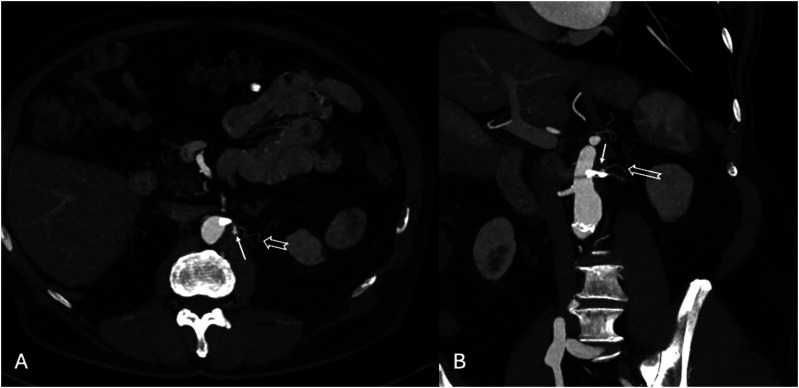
Pre-discharge CTA showing type II endoleak (white arrow) from a 1 mm phrenic artery (empty arrow). (A) Maximum intensity projection (MIP) axial CTA (B) MIP MPR CTA in an obliqual coronal plane.
